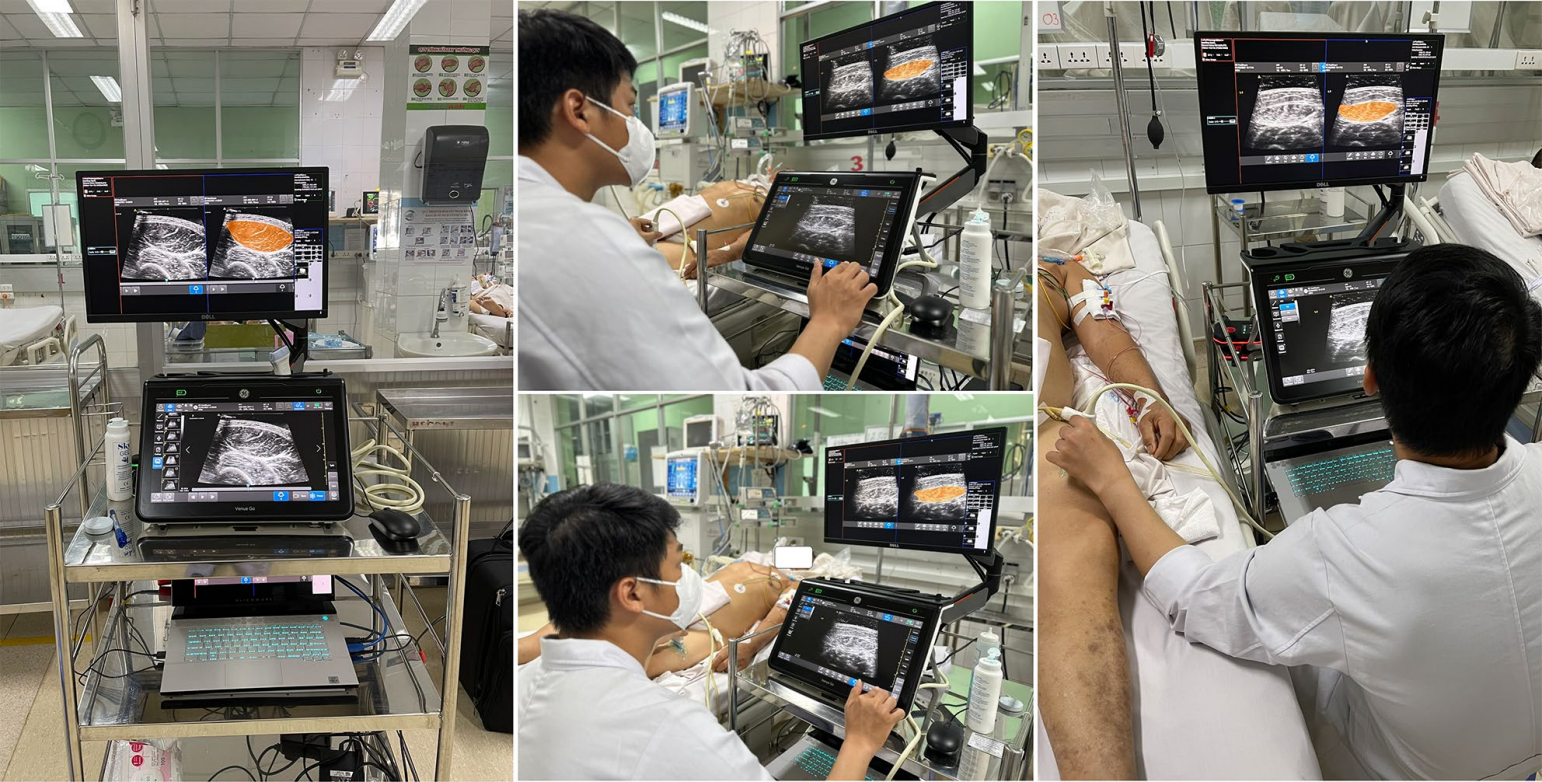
Real-time AI-assisted muscle ultrasound (RAIMUS) system.
